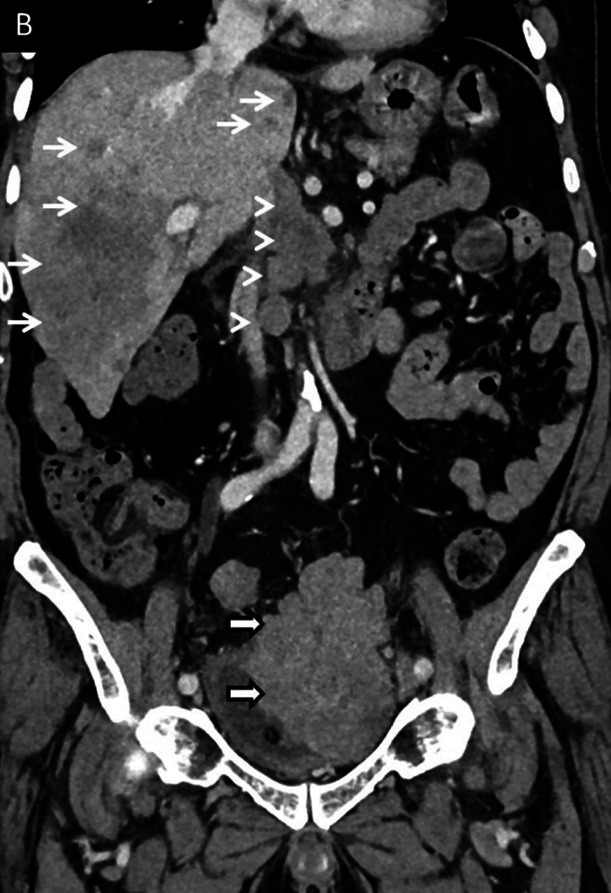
B – Coronal contrast-enhanced CT images arterial phase showing lobulated enhancing bladder mass (thick arrows), venous phase CT images showing paravesical extension (black arrows) with bulky retroperitoneal and abdominal lymphadenopathy (arrowheads) and multiple liver metastases (white arrows).
